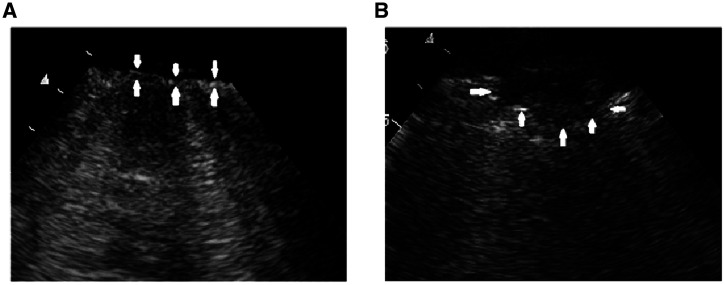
Pleural line abnormalities (A) and subpleural consolidation (B) identified with lung ultrasound in patient with COVID-19 infection.
